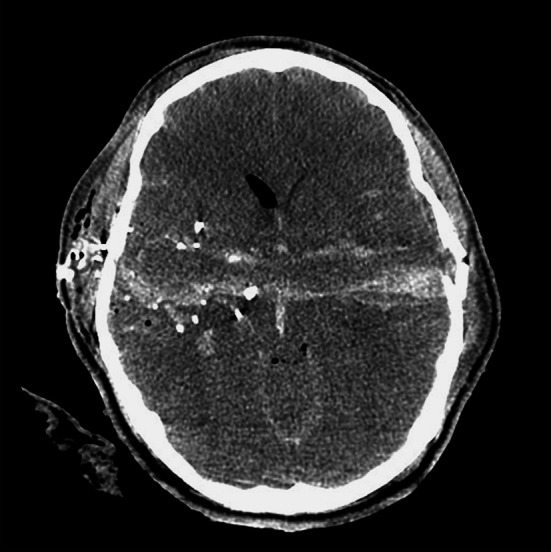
Axial CT scan showing the ‘tram track sign’. The bullet has traversed the cerebral hemispheres with streaks of blood on either side of the low density track. This resulted in a fatal outcome for the patient
